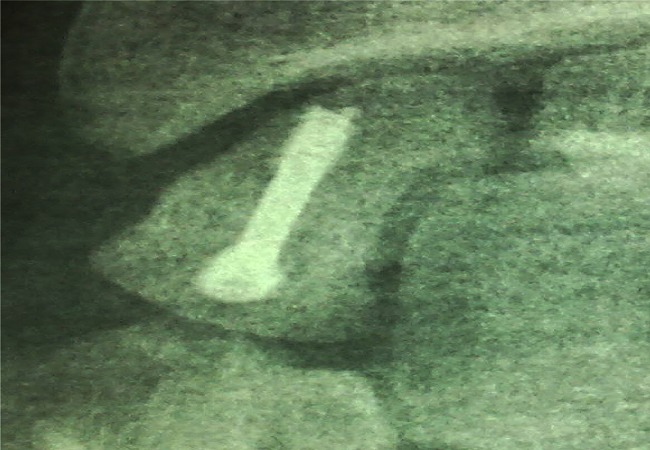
Radiographie de contrôle à la 12ème semaine post-opératoire chez le même patient
